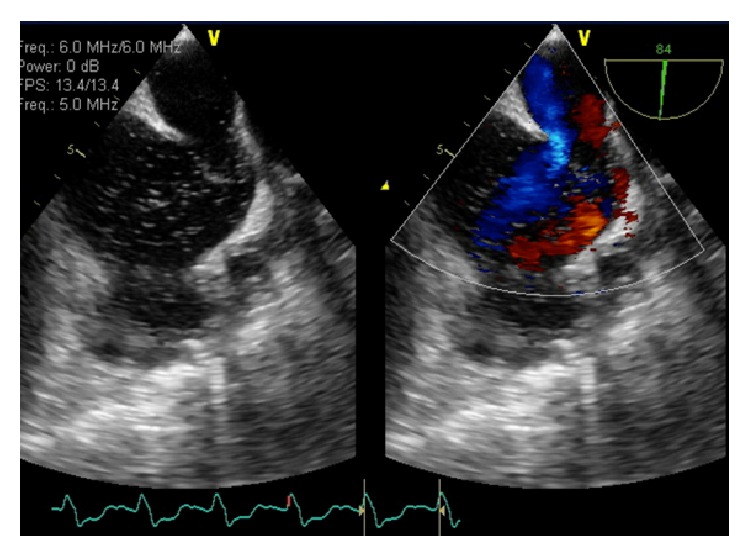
Color Doppler displaying sinus venosus defect.
